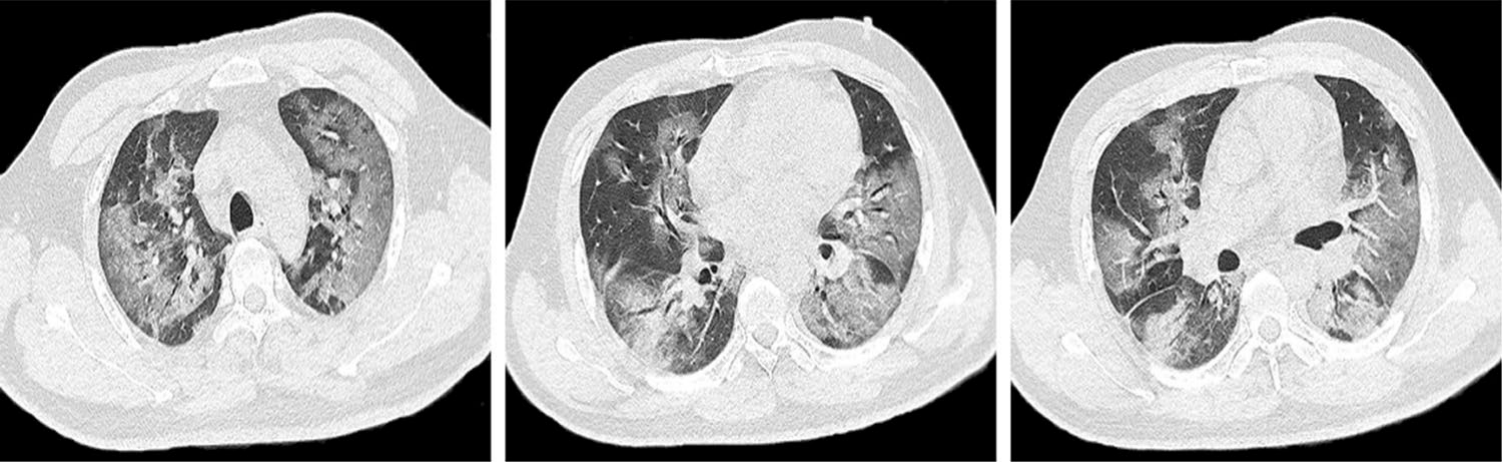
Chest CT feature the multiple lung segments involved. Female, 59 years, confirmed as COVID-19, chest CT images showed crazy-paving pattern and multiple lung segments involved
